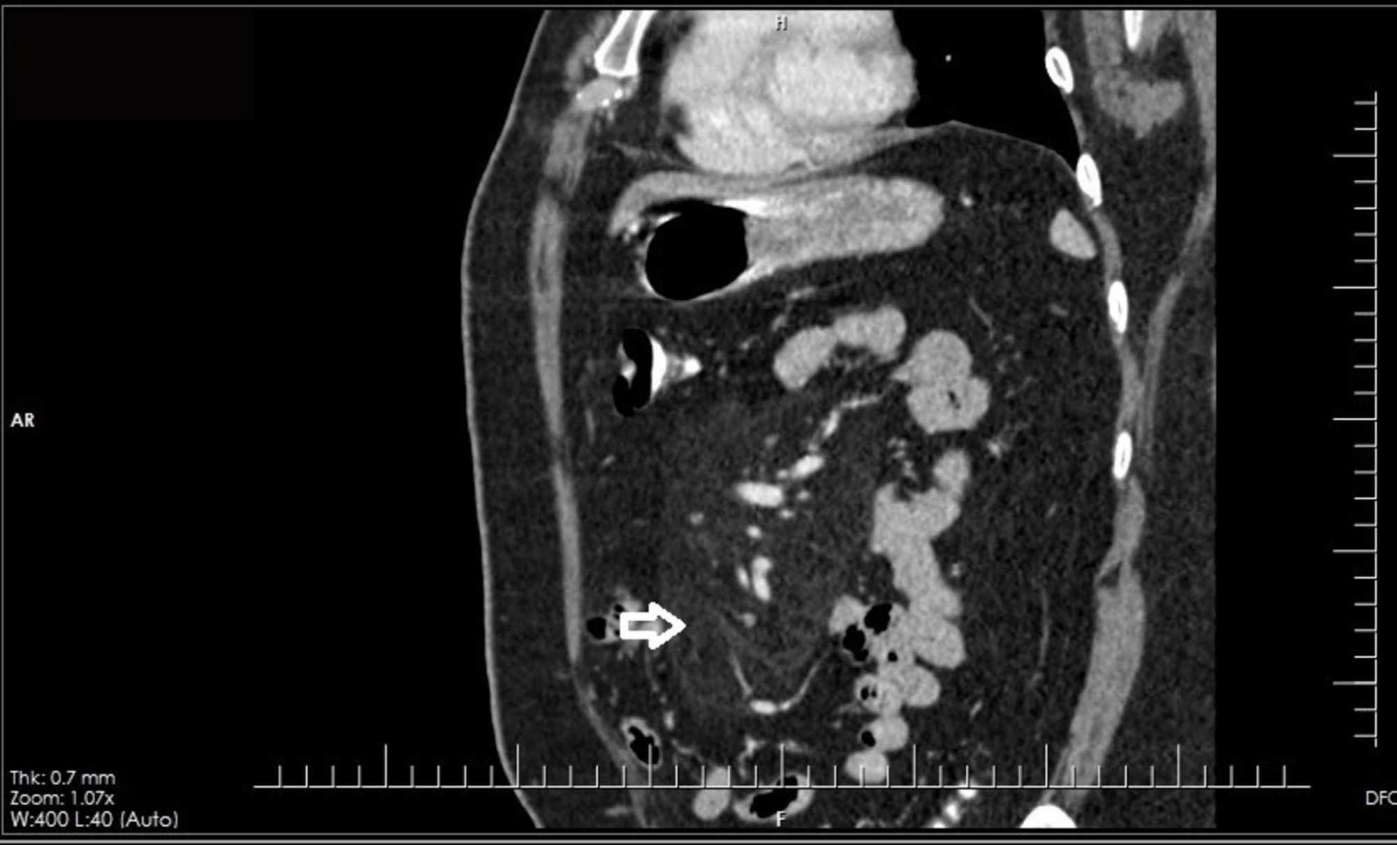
CT sagittal view showing ill-defined, mesenteric fat stranding in the center of the abdomen – in the umbilical and supraumbilical locations and encasing the vessels
